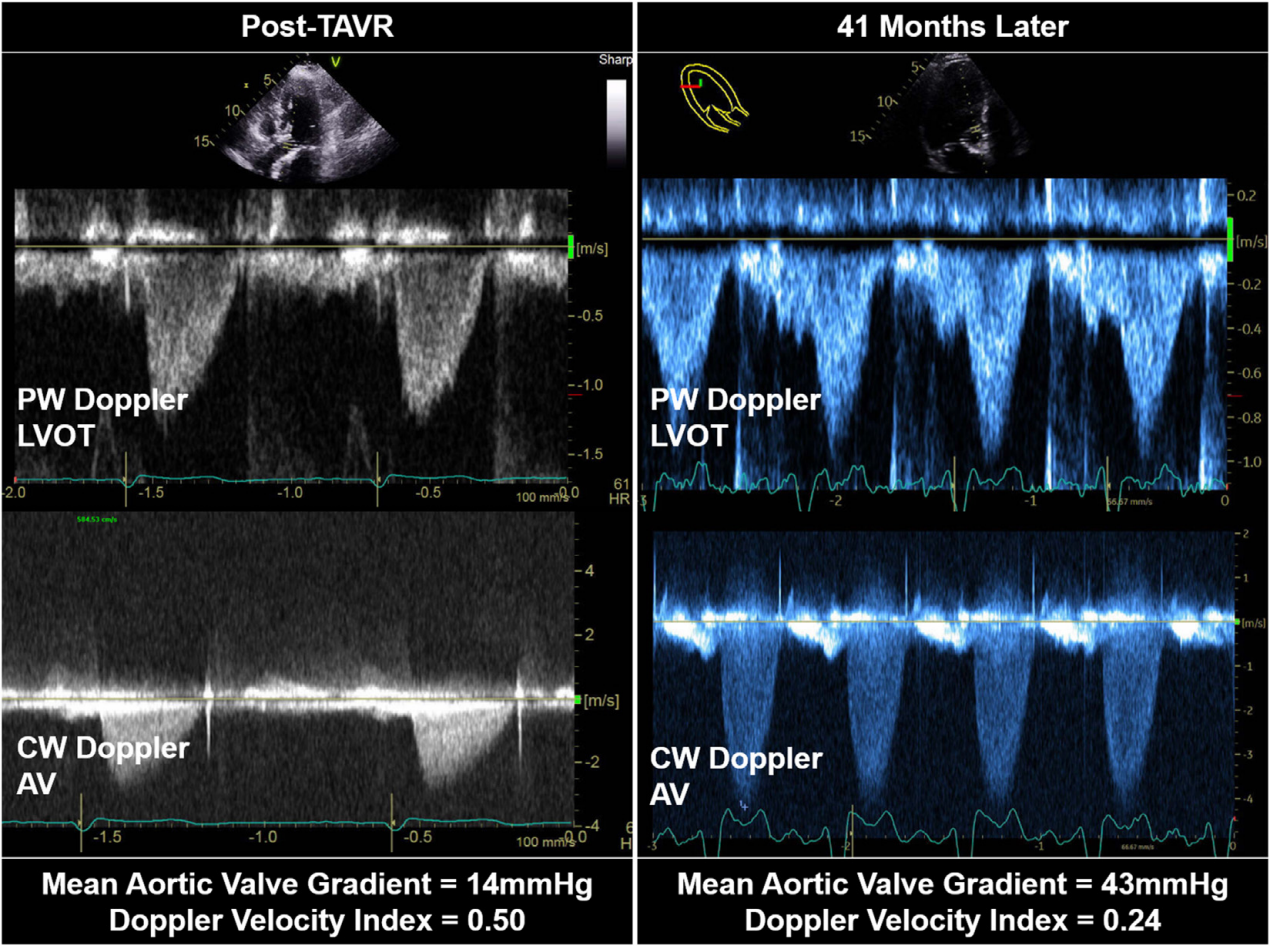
Transcatheter Aortic Valve Doppler Echocardiography Pulsed-wave (PW) Doppler echocardiography of the left ventricular outflow tract (LVOT) and continuous-wave (CW) Doppler echocardiography of the transcatheter aortic valve (AV) demonstrated progression of aortic stenosis 41 months after transcatheter aortic valve replacement (TAVR).
